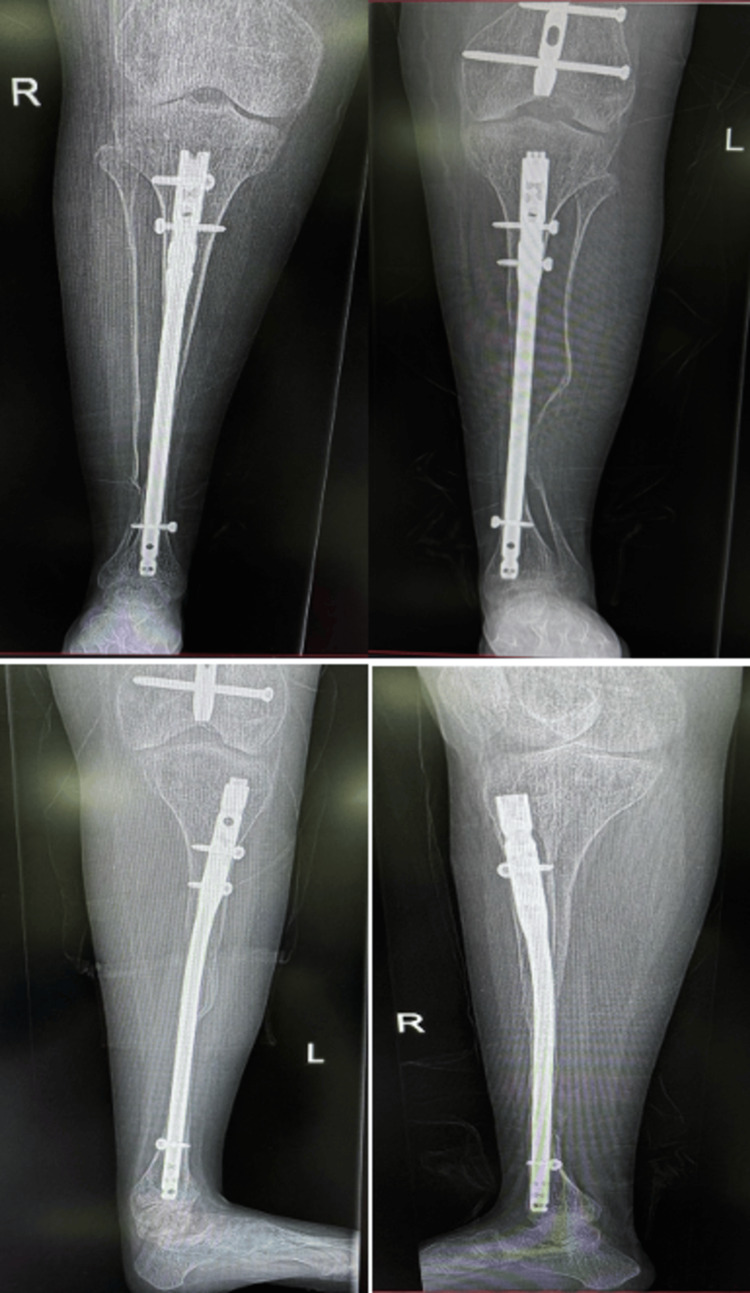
X-rays six months postoperatively Six-month follow-up radiographs of both tibiae in anteroposterior and lateral views showing maintained alignment and stable intramedullary nail fixation following corrective osteotomies. Evidence of satisfactory healing is noted without signs of implant loosening or displacement.
